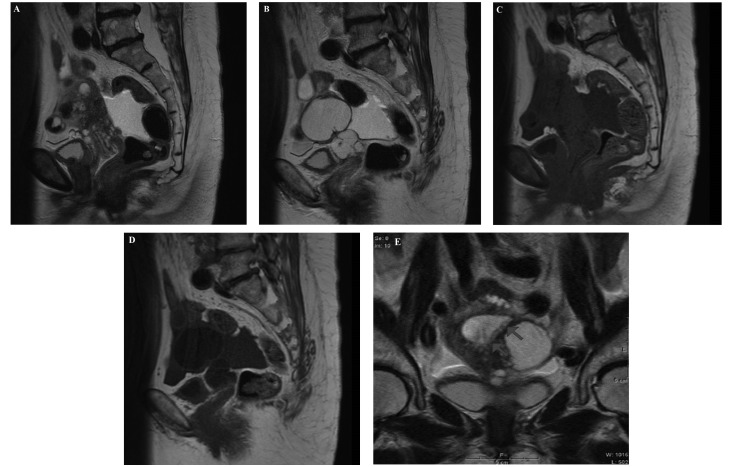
(A) T2-weighted sagittal magnetic resonance imaging revealing (A) no evident enlargement of the uterine cervix and (B) several high intensity cystic lesions in the uterine corpus and uterine cervix. (C) T1-weighted sagittal magnetic resonance image corresponding to Fig 2A. (D) T1-weighted sagittal magnetic resonance image corresponding to Fig. 2B. (E) T2-weighted coronal magnetic resonance image showing thickening of the endometrium (arrow).
